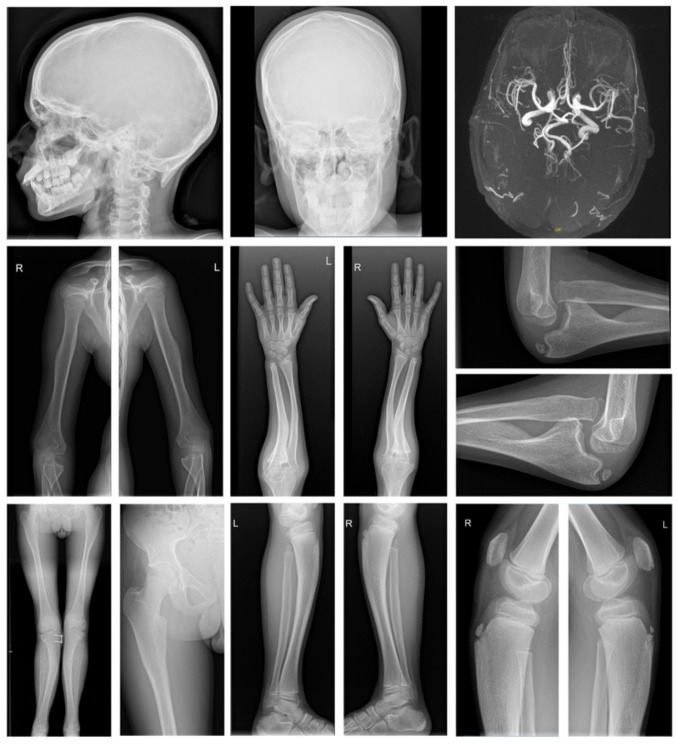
Total skeletal radiography and MRI/MRA studies in subject F1:II-1 showing severe skeletal dysplasia with occipital horns, undertubulation of bony structures and broadening of the ventral end of the first left rib, both claviculae and scapular neck, bowing of the long bones with mid-diaphyseal broadening, bilateral luxation of the radial heads, bilateral overgrowth of the ulnar coronoid processes, prominent trochanter minor of both femurs, short fibula, bilateral coxa valga with rounded iliac wings and broad metatarsals. Brain MRI and angiography showed tortuous intracranial arteries and normal brain structure.
